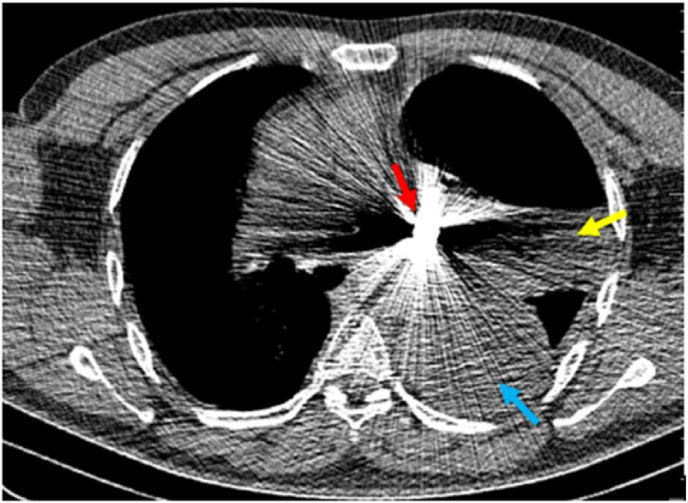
Non-contrast CT chest demonstrating impacted foreign body (Bullet) (red arrow) in left hemithorax especially left main bronchus with resultant left lung atelectasis (yellow arrow) and thoracic empyema (blue arrow). (For interpretation of the references to colour in this figure legend, the reader is referred to the Web version of this article.)
